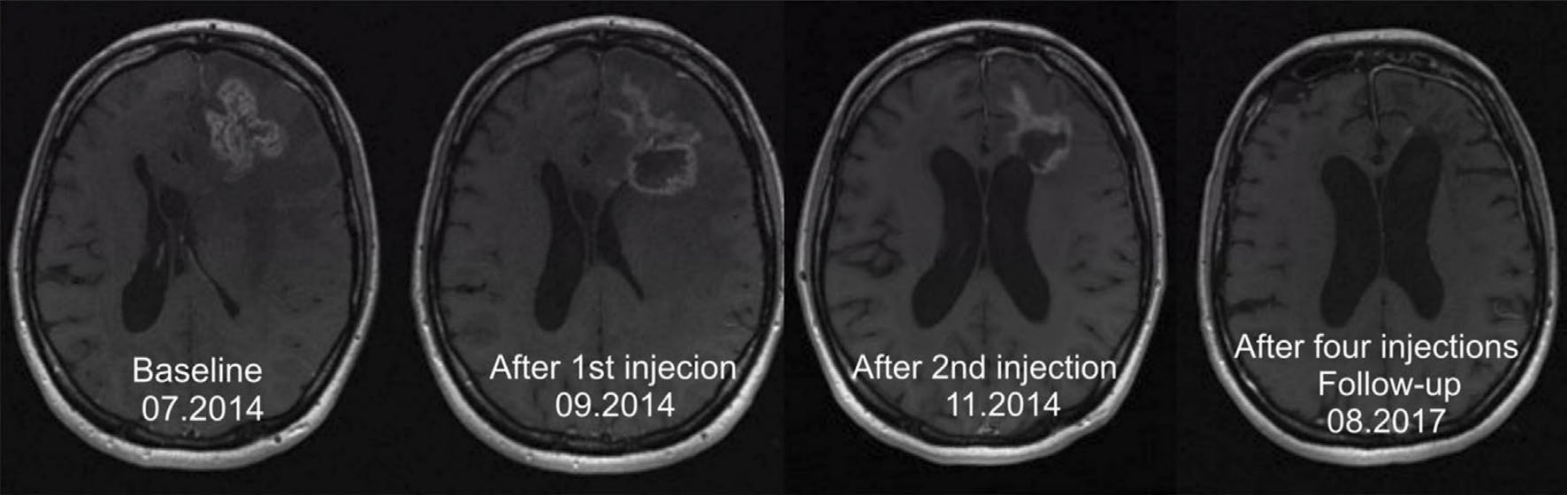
In a 32-year-old woman suffering from an astrocytoma WHO grade II, conversion into a secondary Glioblastoma multiforme (GBM) manifested 10.6 months after initial diagnosis. Following standard treatment consisting of surgery, radiotherapy, and chemotherapy with temozolomide, four cycles of ^213^Bi-DOTA-substance P were applied. The total activity injected amounted to 8.0 GBq of the therapeutic isotope. The T1-weighted enhanced MRI examination revealed shrinkage of the tumor by 32% (Krolicki et al., 2018).
